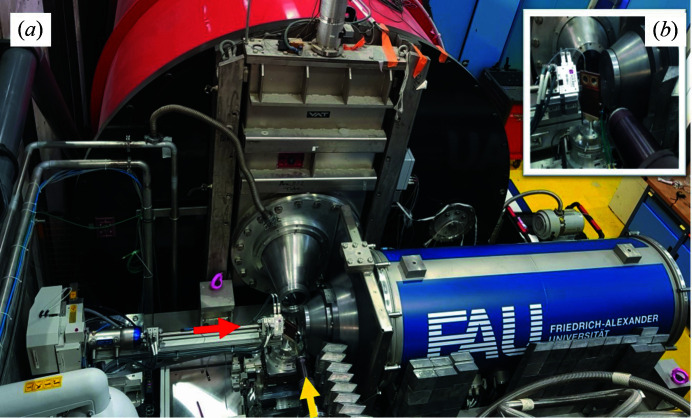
(*a*) Photograph of the SAXS instrument installed at the D22 instrument (ILL) for simultaneous SAXS/SANS experiment. (*b*) A sample at an angle of 45° relative to both orthogonal neutron and X-ray beams. Lead shielding can be seen (1) on the front of the detector vacuum chamber, (2) along the neutron collimation and (3) on the side of the vacuum chamber of the X-ray detector. The red and yellow arrows indicate the directions of the X-ray and neutron beams, respectively.
